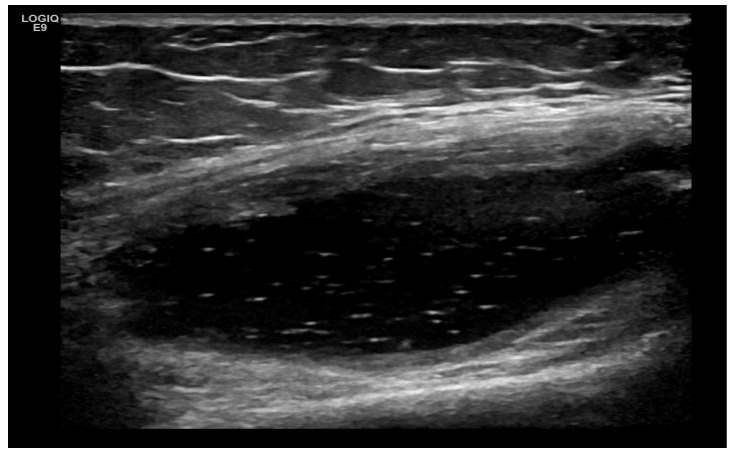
Ultrasound image of the so-called “starry sky” or “snowstorm” appearance in a suprapatellar recess in a patient with OA. Against the background of the anechoic US image of the hydrops, the hyperechoic particles in the middle of the liquid stand out well, which correspond to formed crystalline substances, established in crystalline arthropathy from monosodium urate.
